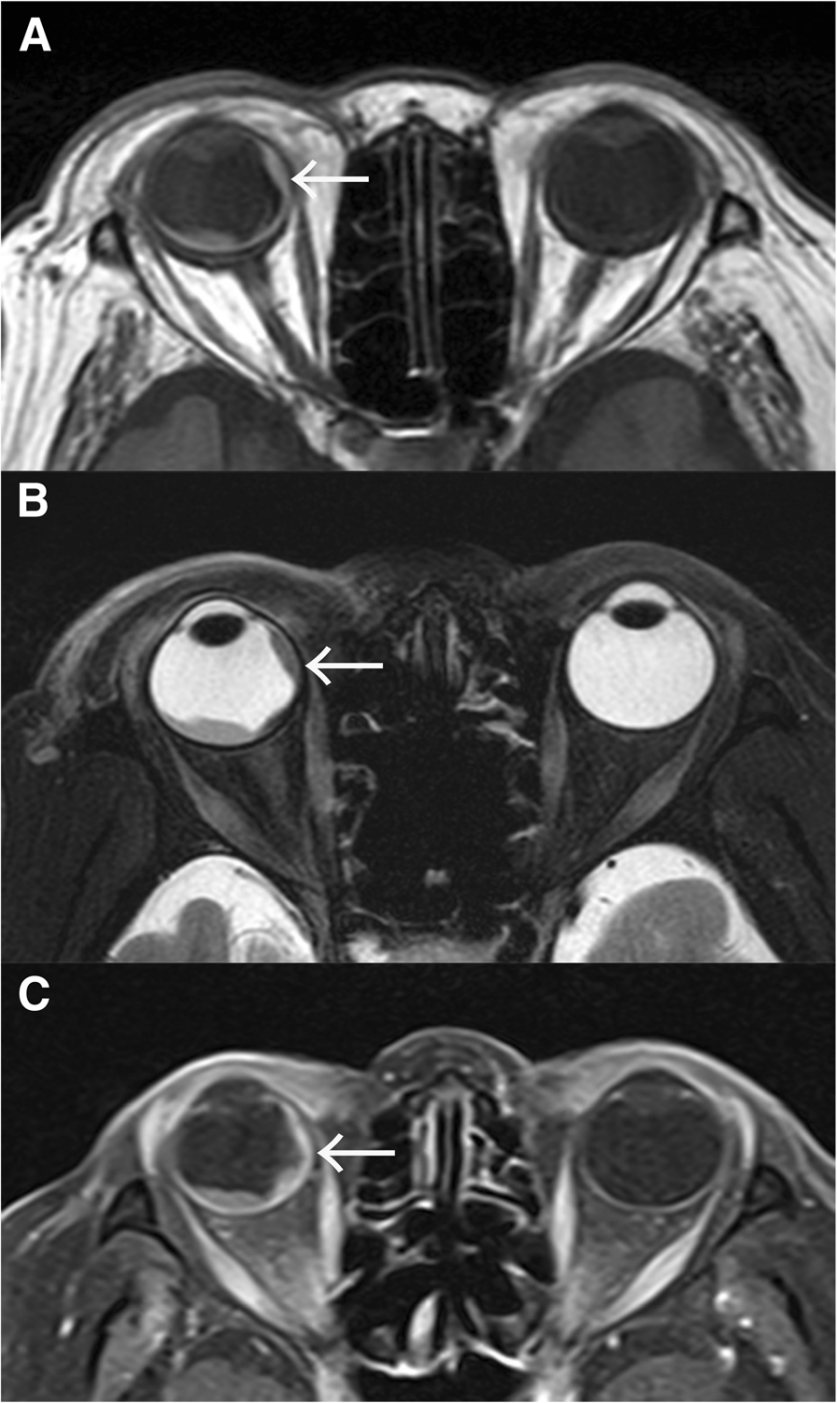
T1-weighted (a), T2-weighted (b), and gadolinium-enhanced (c) magnetic resonance images (MRIs) obtained before enucleation. a T1-weighted MRI displays relatively high intensity at the site of the tumor (*arrow*), whereas retinal detachment reveals isointensity in the inferior retina. b T2-weighted MRI displays low intensity in the nasal retina where the choroidal tumor situates (*arrow*). c The tumor is enhanced by gadolinium (arrow)
